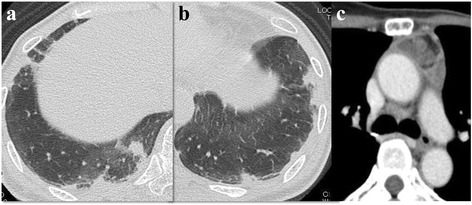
Chest computed tomography scans on admission. a, b Irregular shaped peripheral consolidations in both the lower lobes. c A heterogeneously enhanced mediastinal mass
